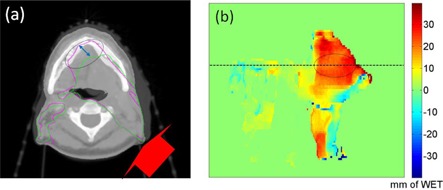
Blended view (a) of the pCT with cCBCT from week 3. The red arrow indicates the direction of the posterior oblique proton beam and the green and magenta contours are the 90% isodose lines for the pCT and cCBCT, respectively. The 2D display (b) of the distal WET difference between the original and new 90% isodose lines in the beam's eye view. The horizontal line indicates the axial slice position of the CT image in (a). Color scale bar is in mm.
